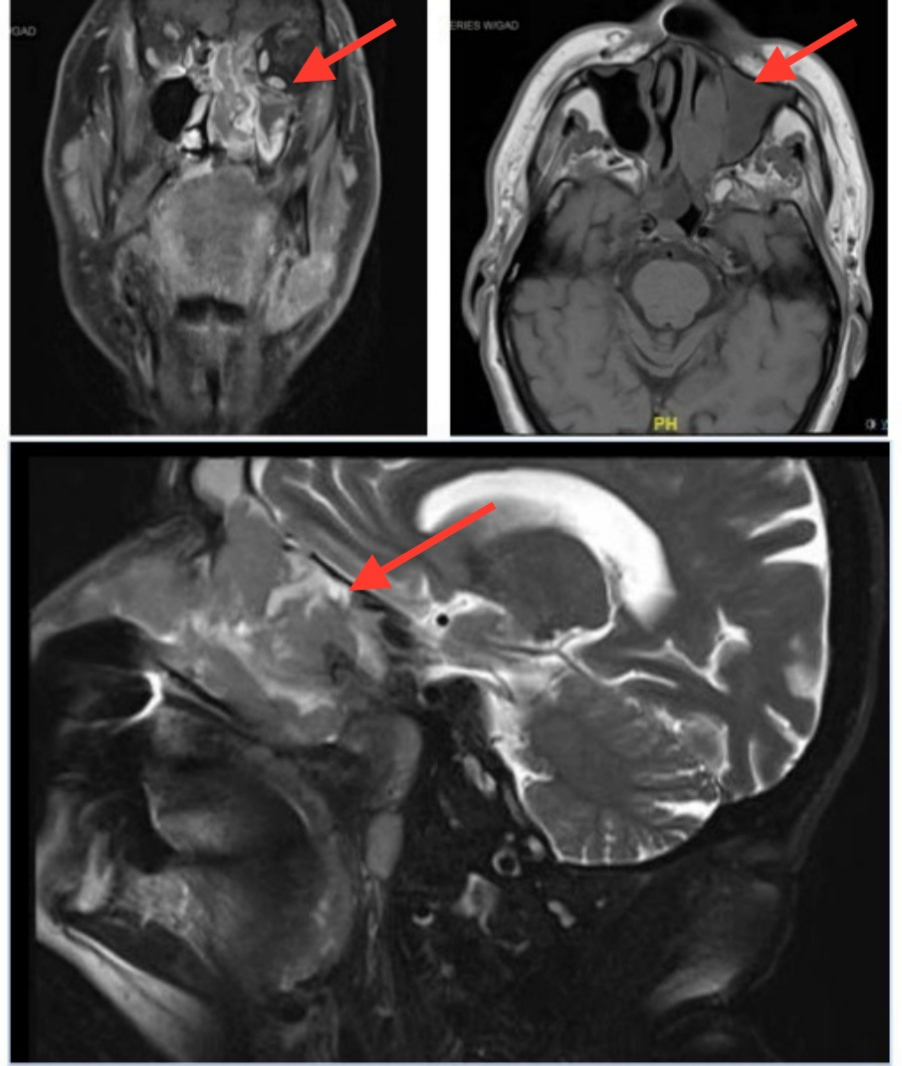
Pre-treatment magnetic resonance imaging (MRI) Clockwise from top left: coronal T1 with gadolinium, axial T1, and sagittal T2
